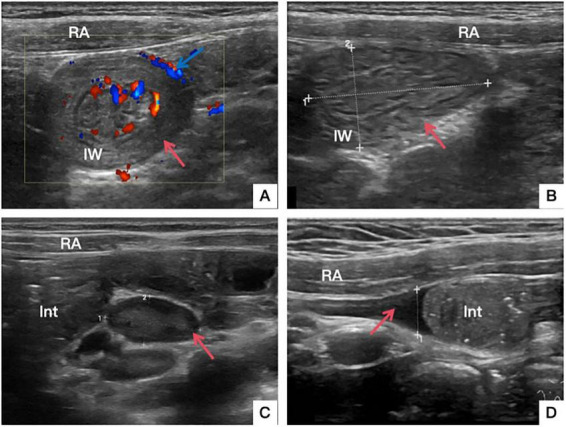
Ultrasound findings. (A) Red arrow: “target sign”; Blue arrow: blood flow in the intestinal wall. (B) “Kidney sign”. (C) Lymph nodes. (D) Ascites. RA, rectus abdominis; Int, intestine; IW, intestinal wall.
